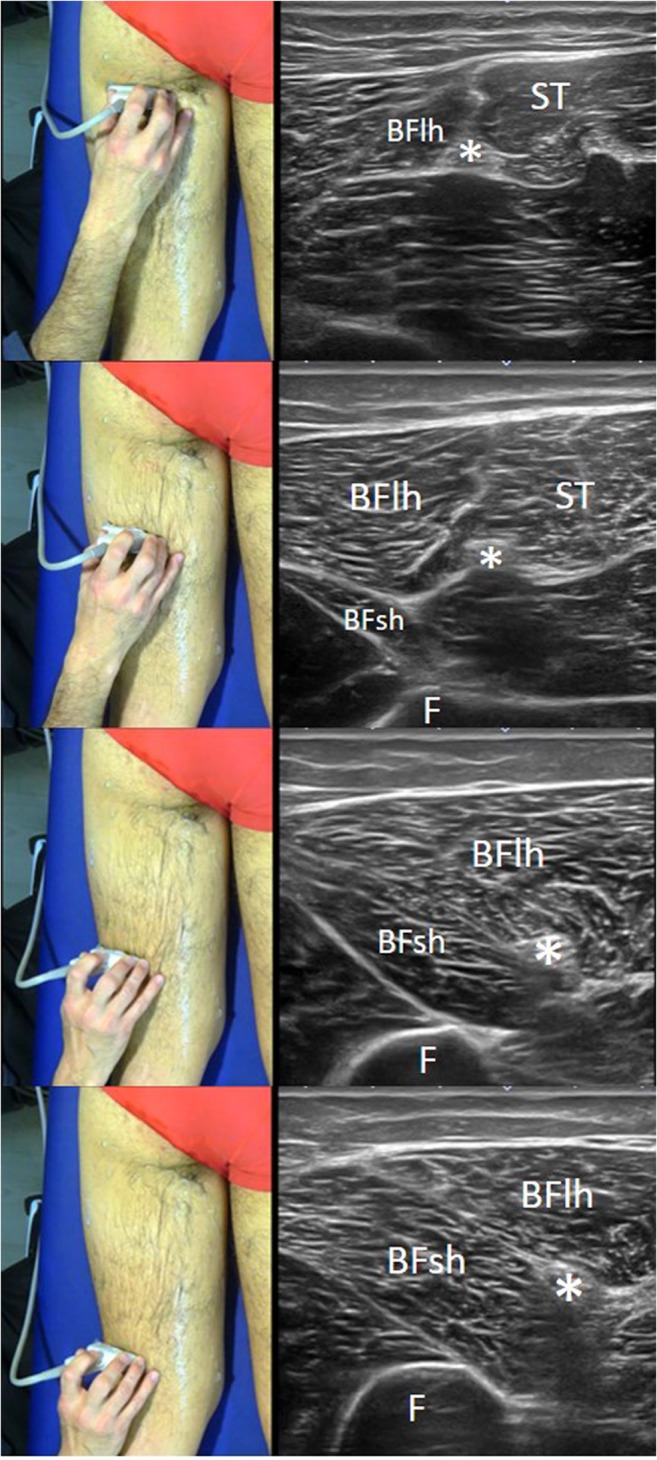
Series of short-axis ultrasound scans showing different views of the lateral part of the hamstrings (lateral arm of the tuning fork). As the probe is moved distally along the sciatic nerve pathway, the section of the short head of the biceps femoris muscle can be seen to appear. The photographs on the left of the figure indicate probe positioning. *BFlh* long head of the biceps femoris, *BFsh* short head of the biceps femoris, *ST* semitendinosus muscle, (*) sciatic nerve
